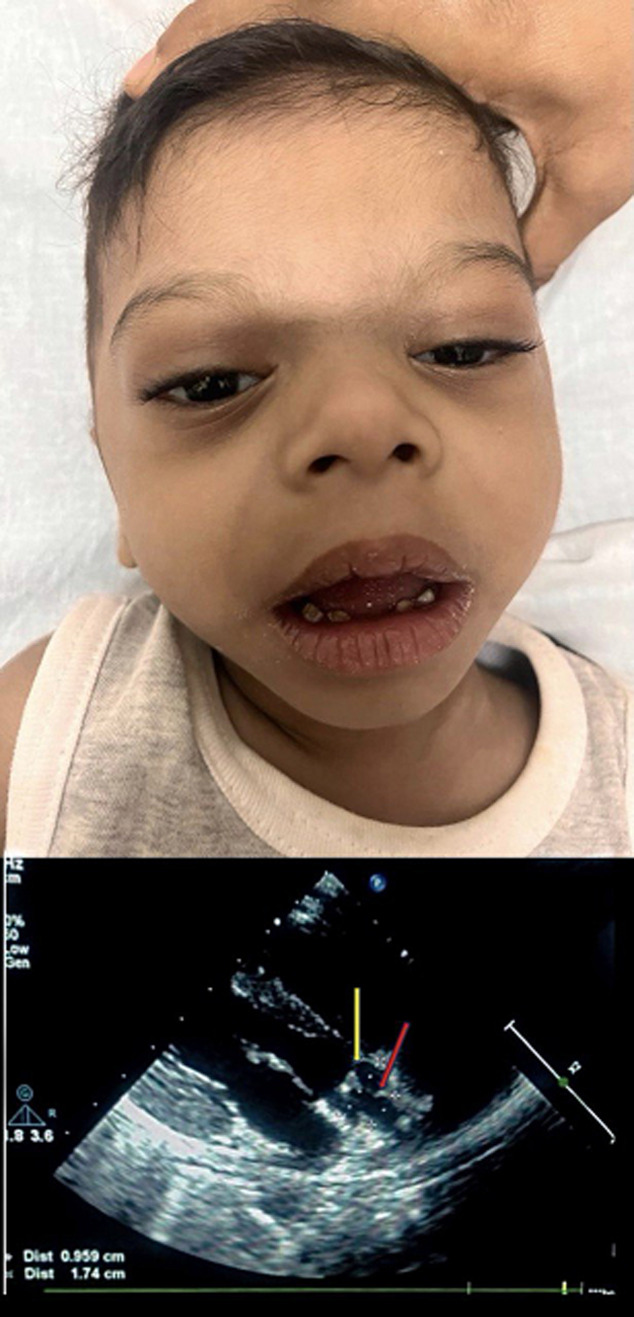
classical elfin facies with 2D echocardiogram (parasternal short axis view) showing supravalvular aortic stenosis (red arrow) and aortic valve (yellow arrow)
